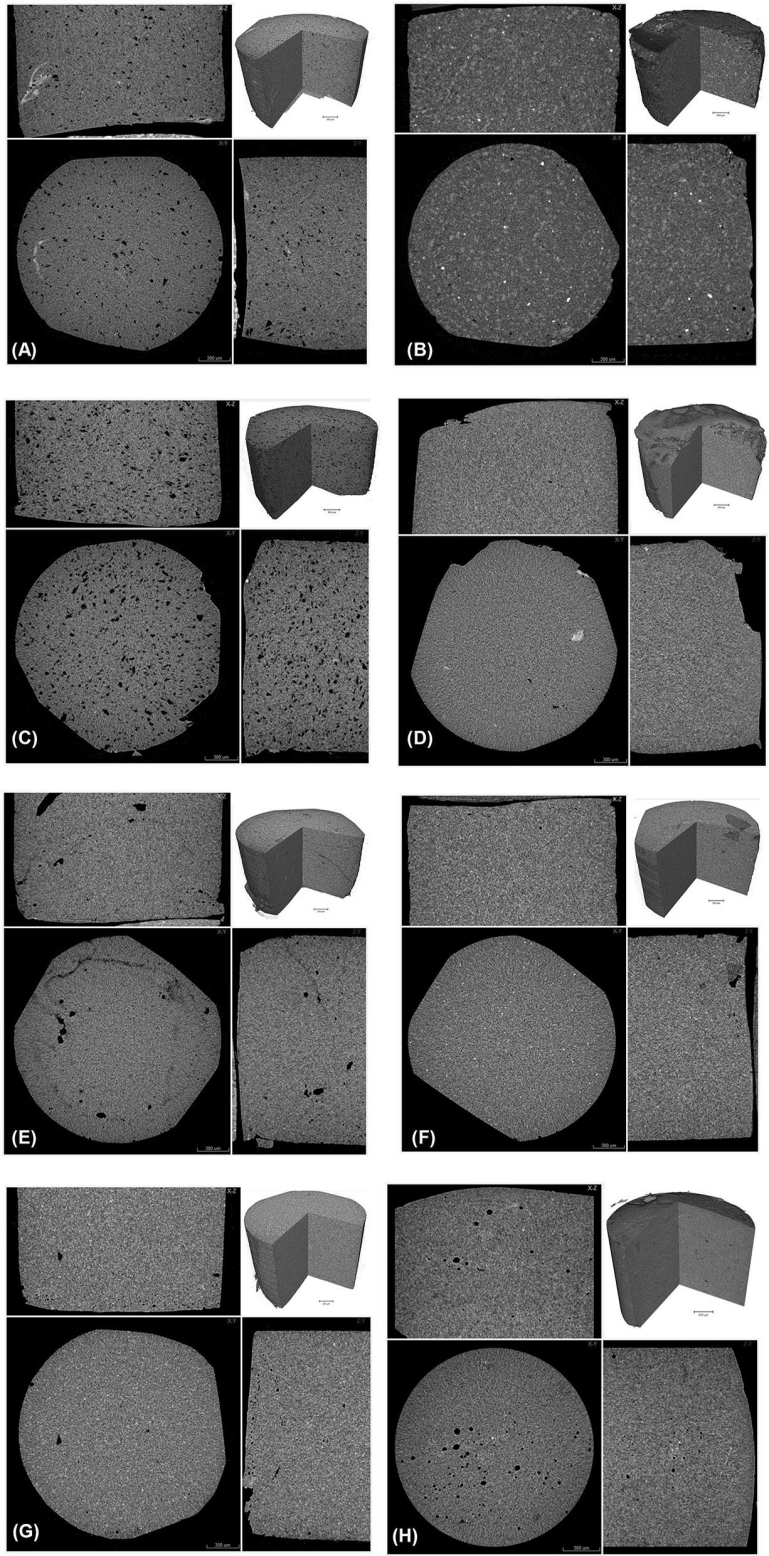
Micro-computed tomography of (A) Charisma, (B) Tetric EvoCeram, (C) Synergy D6, (D) Filtek Supreme XTE, (E) Ceram X, (F) IPS Empress Direct, (G) Grandio nano and (H) Venus. Images show a cross sectional slice scaled at 300 µm with an inset 3D image scaled at 250 µm.
